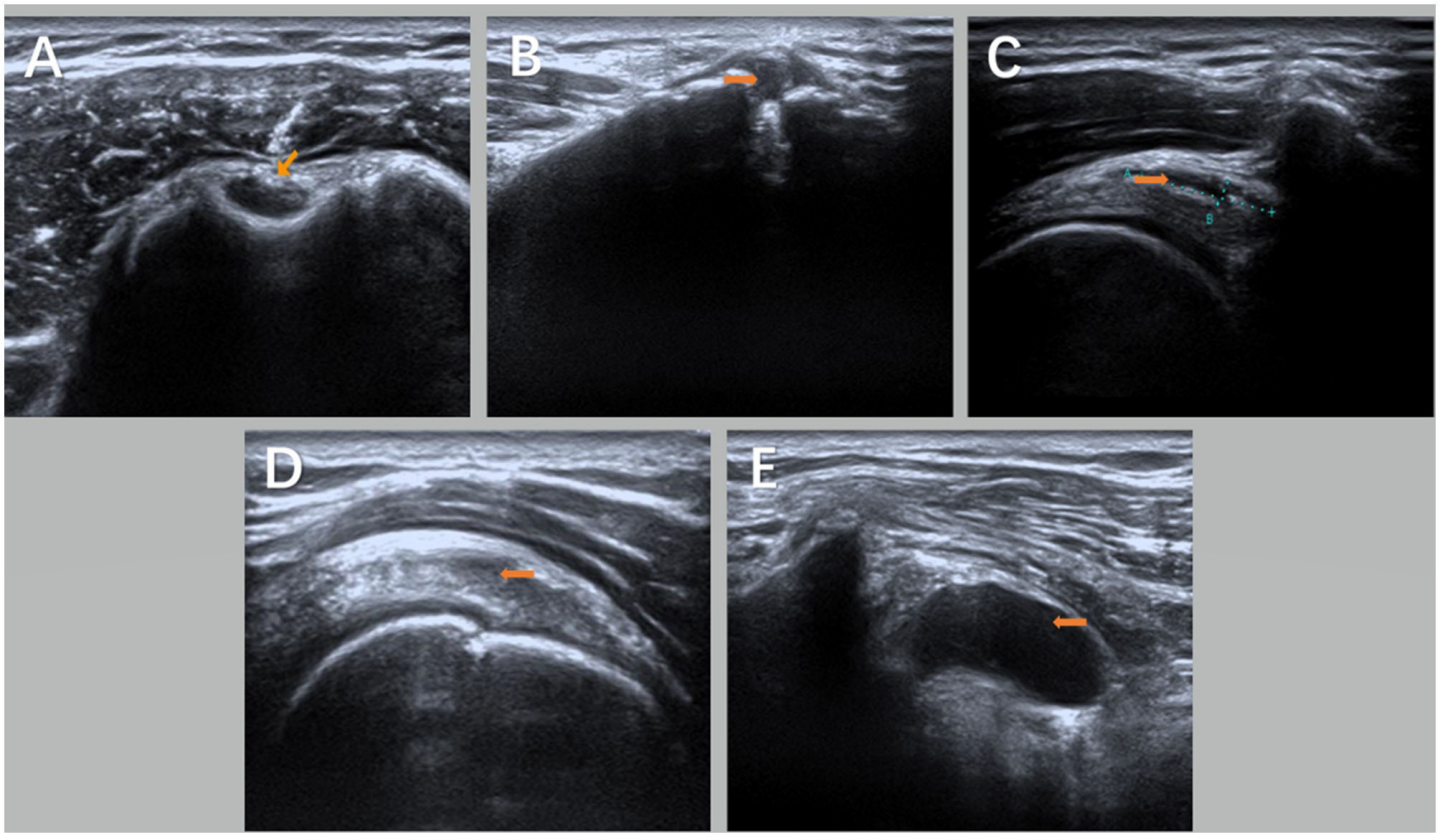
Ultrasound images of the shoulder joint on the hemiplegic side of the subject under the guidance of ultrasound [(A) is biceps long head tendinitis with tendon sheath effusion; (B) is acromioclavicular joint effusion with calcification; (C) is acromion-deltoid muscle Subscapular bursa effusion; (D) is supraspinatus tendinosis; and (E) is coracoid-subscapular bursa effusion]. Orange arrows show the lesion in (A–E).
